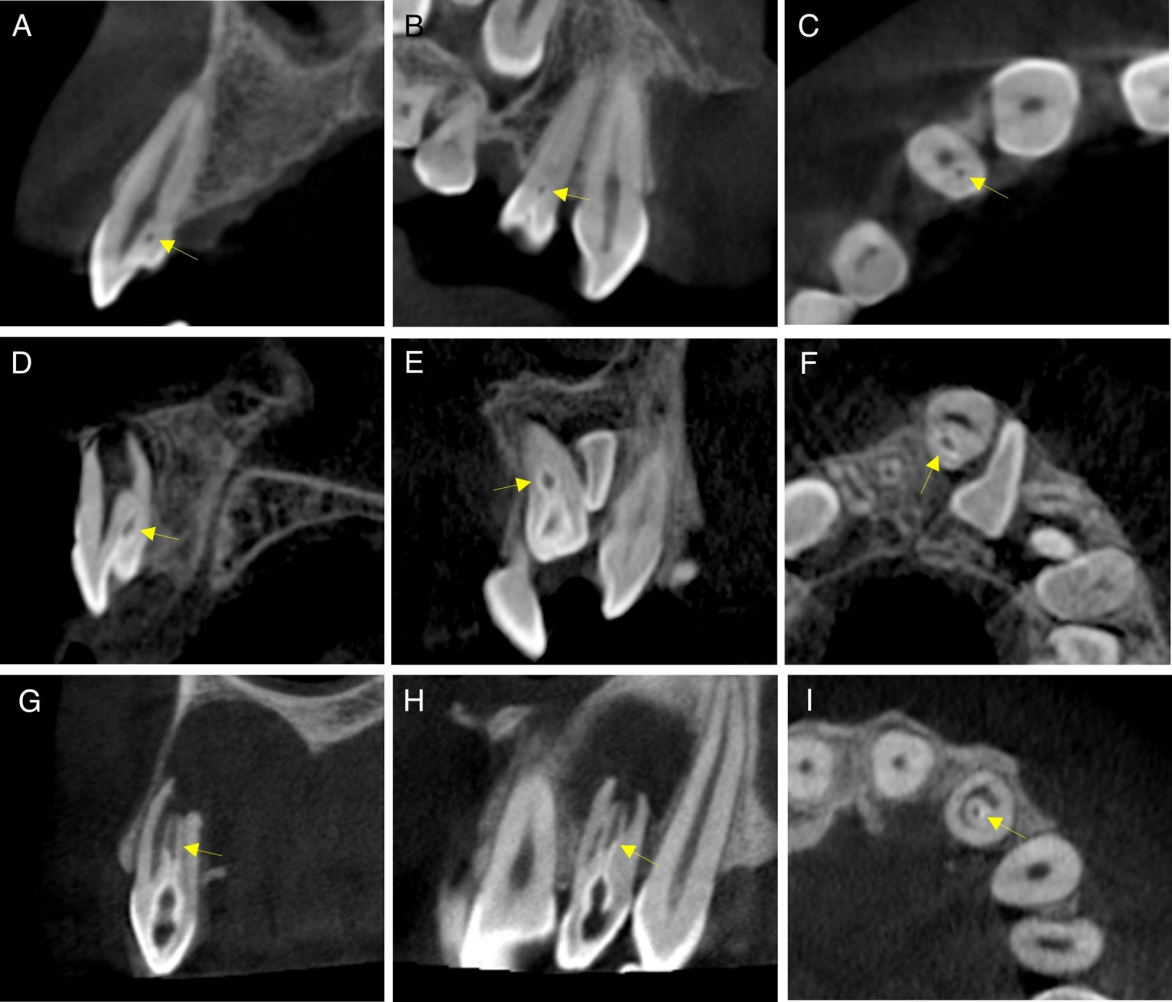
Representative CBCT images of the three DI types (yellow arrows). (a–c) Maxillary lateral incisor with DI type I. (d–f) Mesiodens with DI Type II. (g–i) Maxillary lateral incisor with DI type III. (a, d, g) Sagittal sections. (b, e, h) Coronal sections. (c, f, i) Axial sections.
